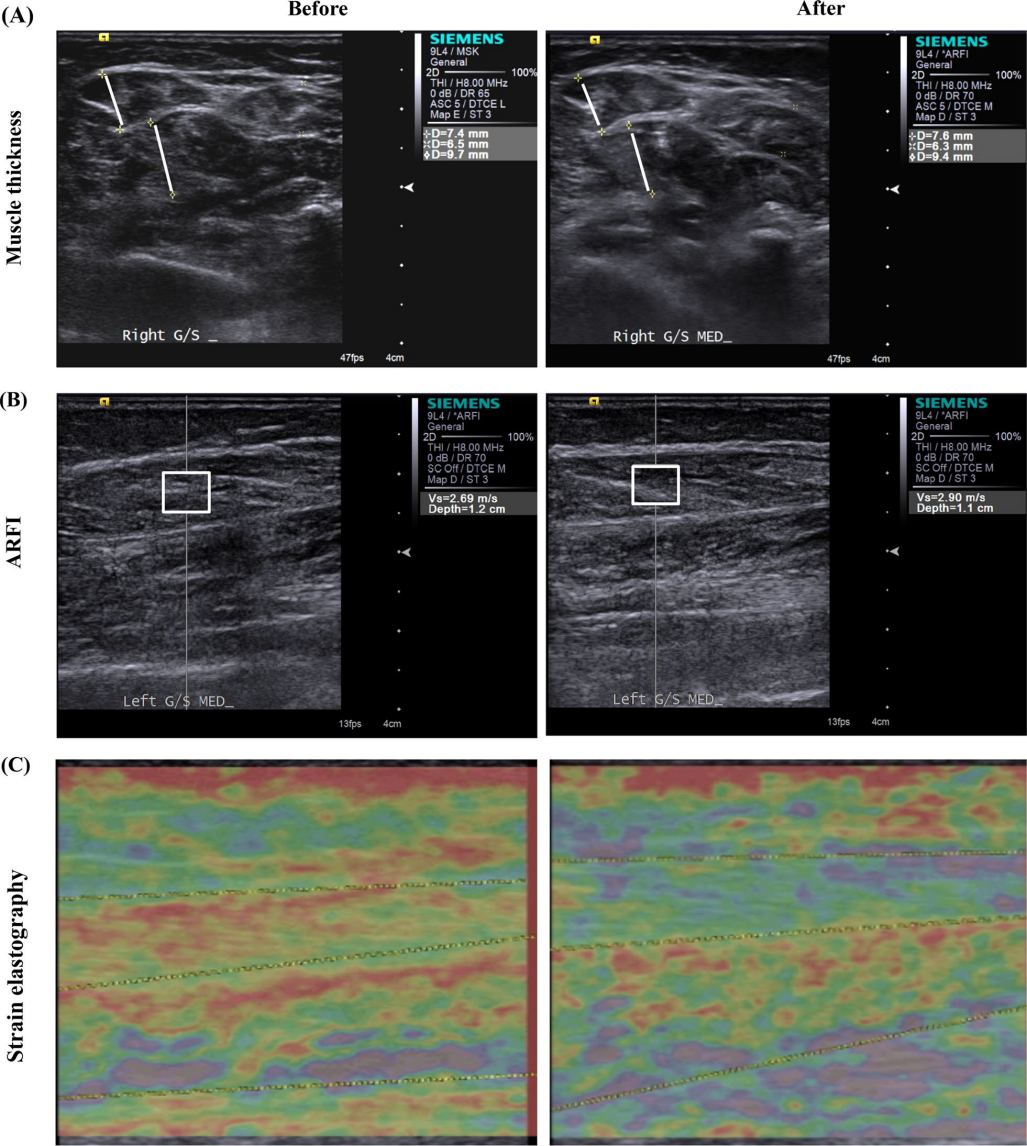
The ultrasonographic images recorded before and after ESWT. A muscle thickness, B ARFI, and C strain elastography (ARFI: Acoustic Radiation Force Impulse)
